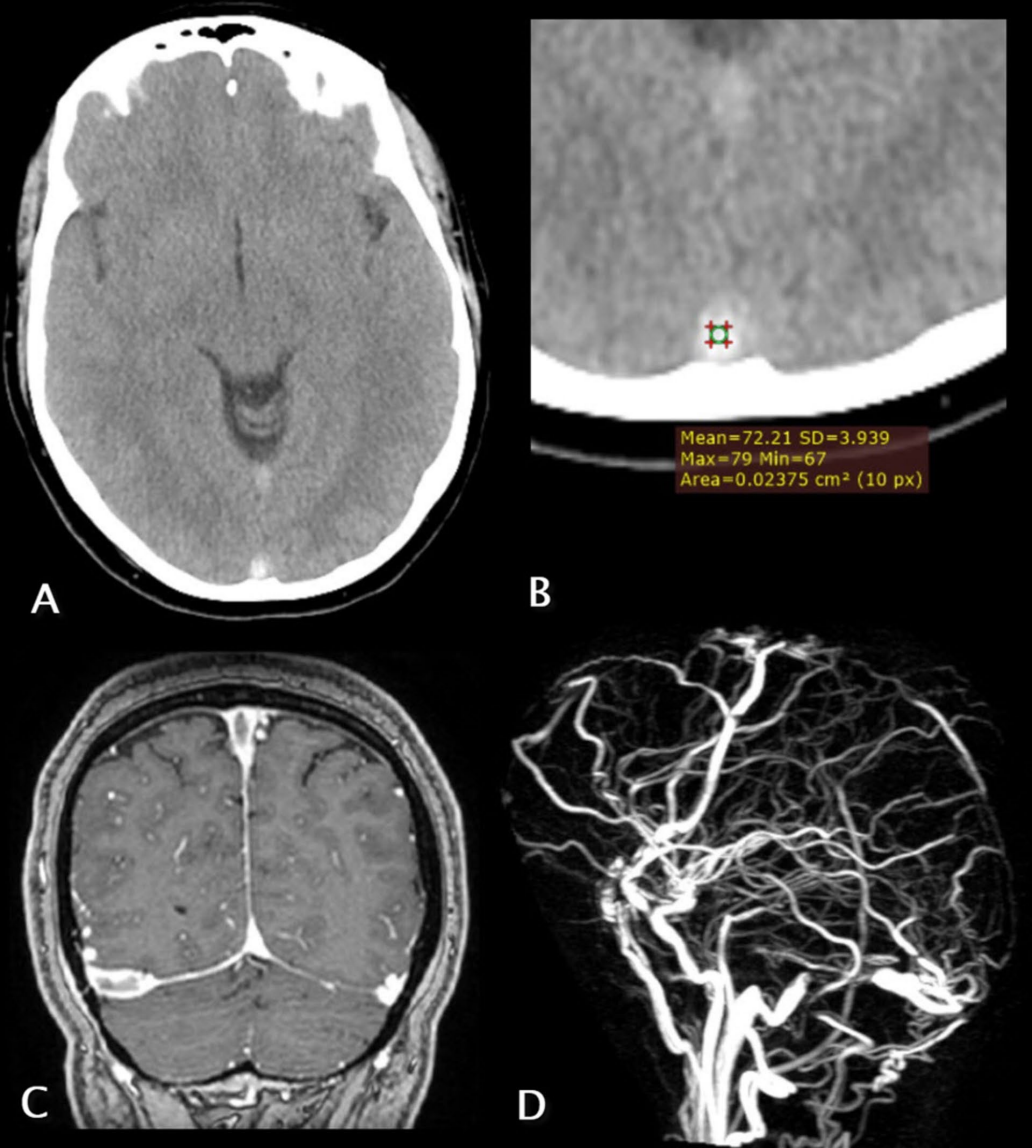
A–D Panels(A) and (B) are axial cuts of a non-contrast CT study of a 37 years old female patient who came to the emergency room complaining of headache and nausea while panels C and D are magnetic resonance images of the patient which were performed on the same day following the CT. Panel (A) revealed a hyperdense inferior segment of the superior sagittal sinus (SSS) and panel (B) shows that the measured density inside the sinus was about 72.4 HU which was significantly high. Panel (C) is a coronal contrast-enhanced MR image which revealed a filling defect at the superior sagittal and right transverse sinuses (empty delta sign) confirming the diagnosis of sinus thrombosis. Panel (D) is 3-dimensional reconstructed MRV image which showed the absence of superior sagittal and right transverse sinus and the presence of numerous tiny crowded vessels in the background presumed to be dilated collateral channels
